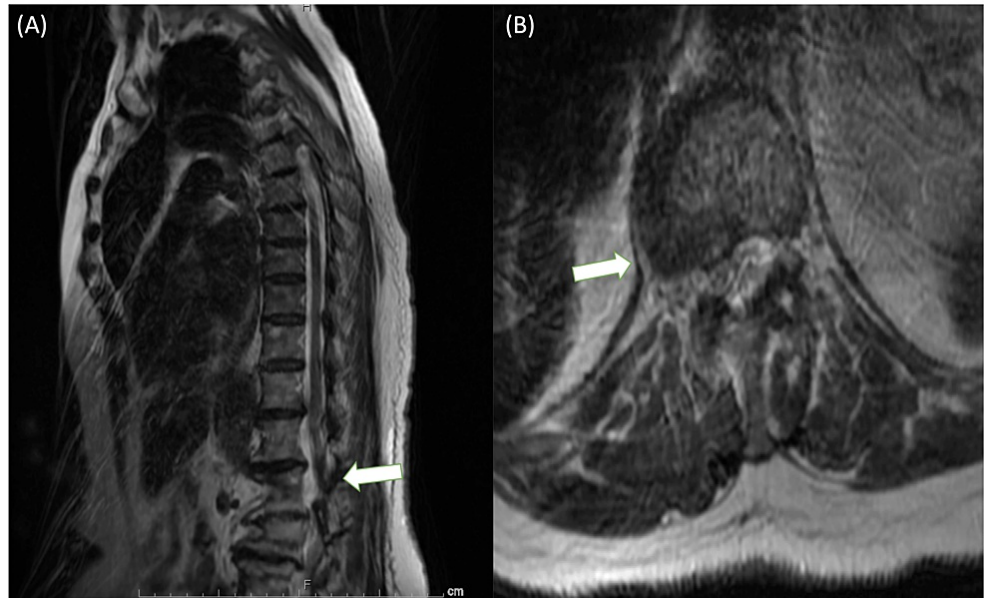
MRI of the thoracic spine (A) Sagittal T2-weighted MRI reveals cord compression at the T12-L1 level. (B) An axial T2-weighted MRI view reveals lateral disc bulging causing cord compression at the T12-L1 level.
